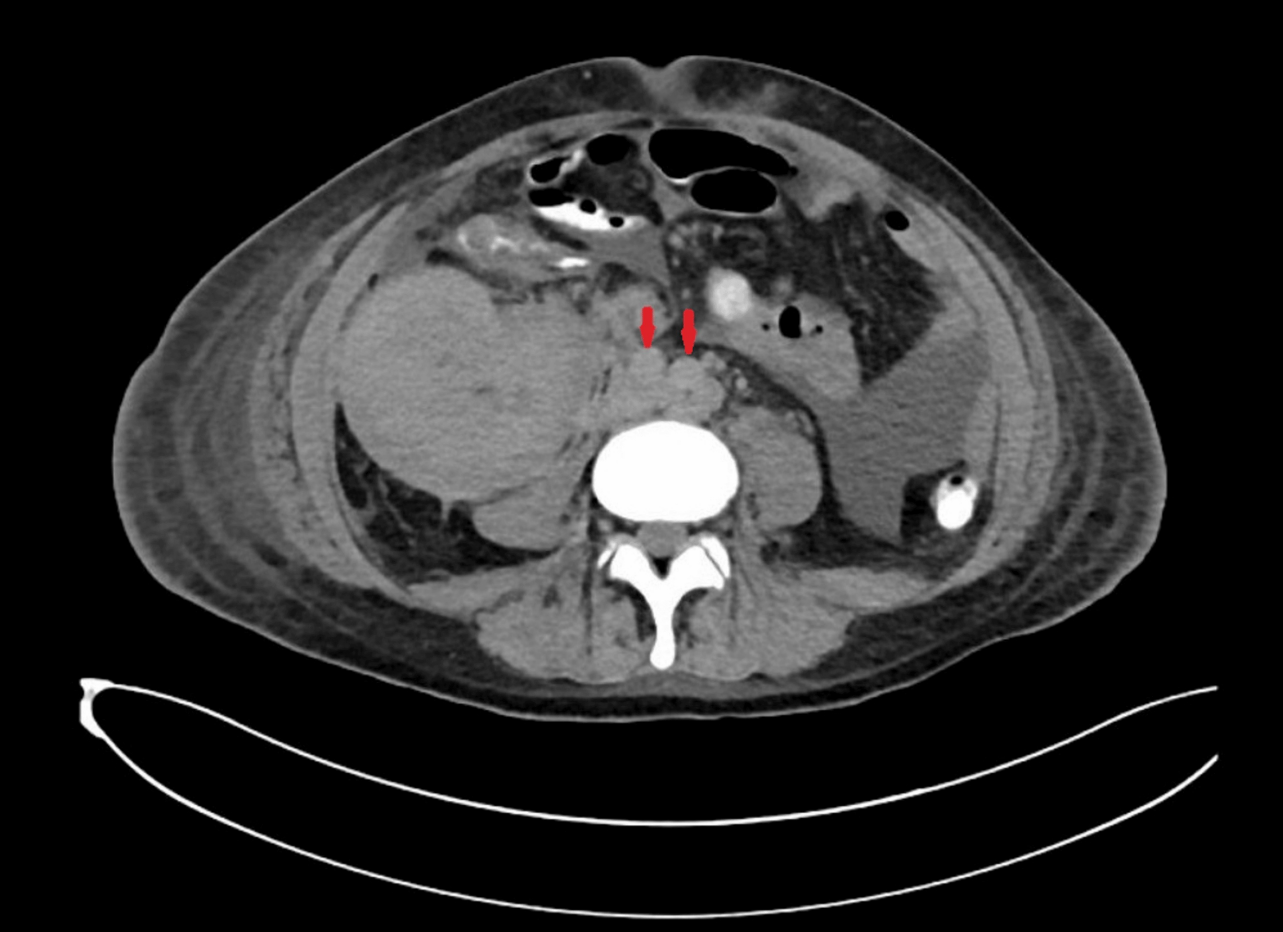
Case 1: CT abdomen The imaging reveals a bulky, non-functioning transplanted kidney exhibiting abnormal enhancement and multiple lymphadenopathies.
